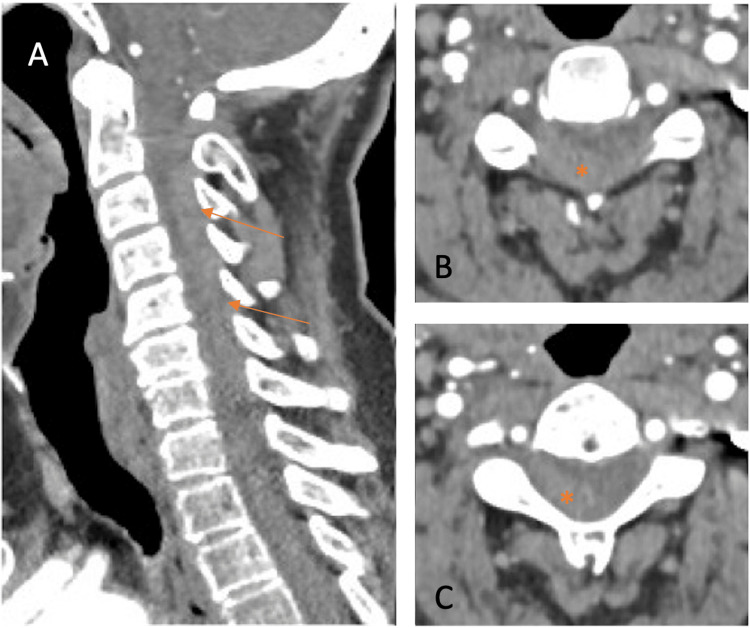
Computed tomography (CT) angiogram of the second case suggesting a cervical spontaneous spinal epidural hematoma (SSEH) (arrows and asterisks) on the right posterolateral side extending from C2 to C7. (A) Sagittal image; (B) axial image at C3; (C) axial image at C5.
